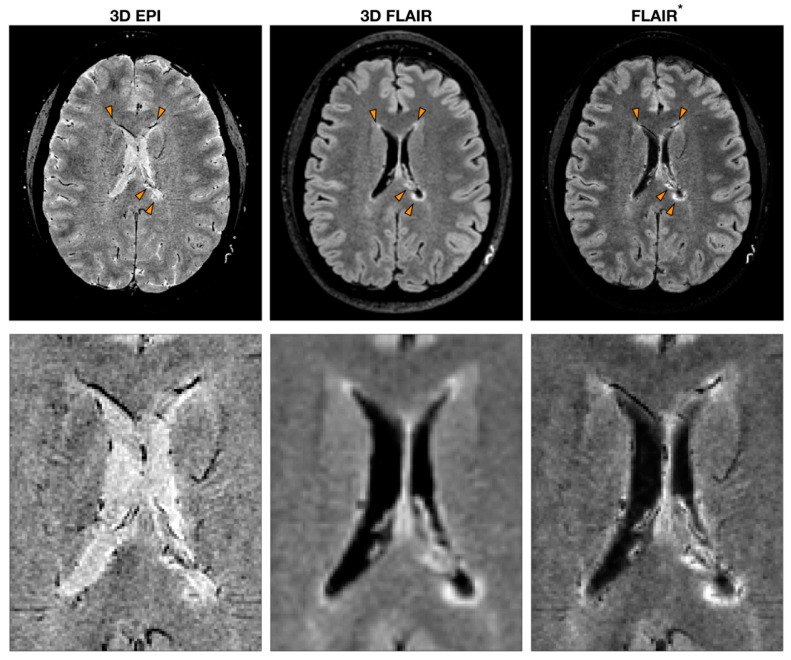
Example of multiple sclerosis (MS) patient MRI images obtained with sequences to detect the central vein sign (CVS). T2*-weighted 3D echo-planar imaging (EPI), 3D fluid-attenuated inversion recovery (FLAIR) and FLAIR*, a post-processing technique that combines the EPI sequence [or more generally a T2*w sequence (used to detect CVS)] and FLAIR. The orange arrowheads indicate MS lesions with the characteristic CVS. A zoomed-in version of the same image is reported to highlight the CVS feature for each modality.
